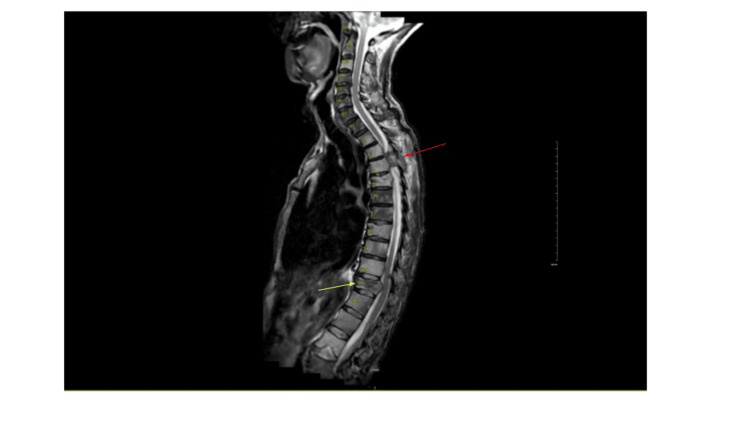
MRI of the spine demonstrating metastatic disease from T3 to T5 (red arrow) causing severe cord compression and a pathologic T11 fracture (yellow arrow)
